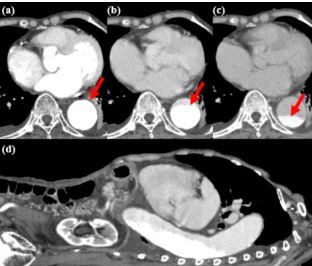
Dynamic, contrast-enhanced computed tomography of the chest and abdomen,demonstrating lessening contrast in aorta over time (arrows): (a) axial image 30 seconds after contrast agent injection; (b) 90 seconds after injection; and (c) 240 seconds after injection; (d) sagittal image at 90 seconds.
